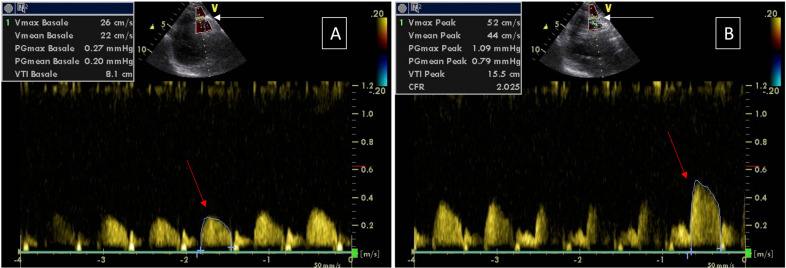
Coronary flow velocity is measured at rest left, Panel (A) and during maximal hyperemia right, Panel (B). In both conditions, pulsed Doppler echocardiography allows to measure the velocity of the blood flow in the distal part of the left anterior descending coronary artery (white arrows). To obtain coronary flow velocity reserve (CFVR), we calculate the ratio between diastolic peak velocity (cm/s) during maximal hyperemia and at rest (red arrows). In this specific patient, CFVR = 52/26 = 2.
